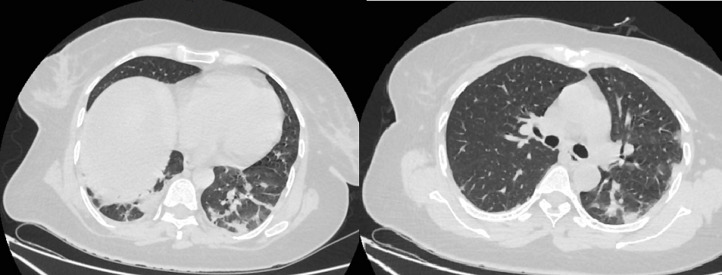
thoracic CT-scan showed lesions compatible with a SARS-CoV-2 infection (ground glass opacities)
